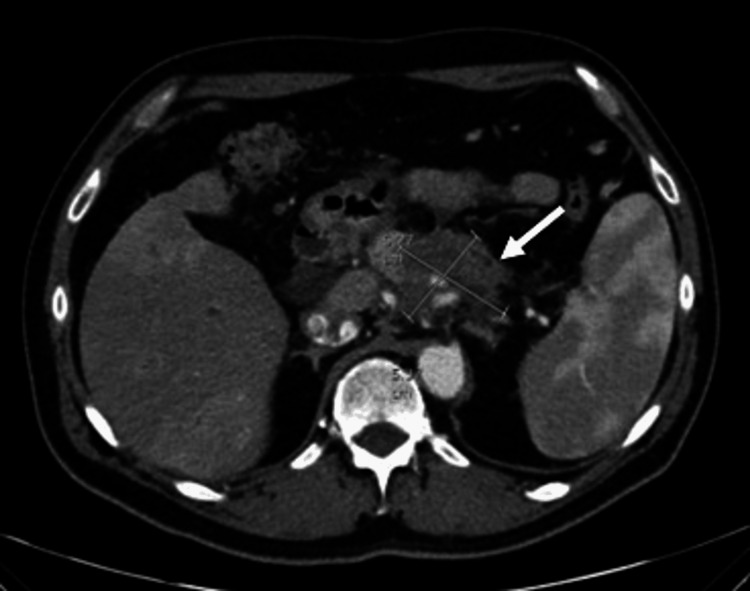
Diagnostic CT showing the patient’s advanced pancreatic tumor CT: Computed tomography
